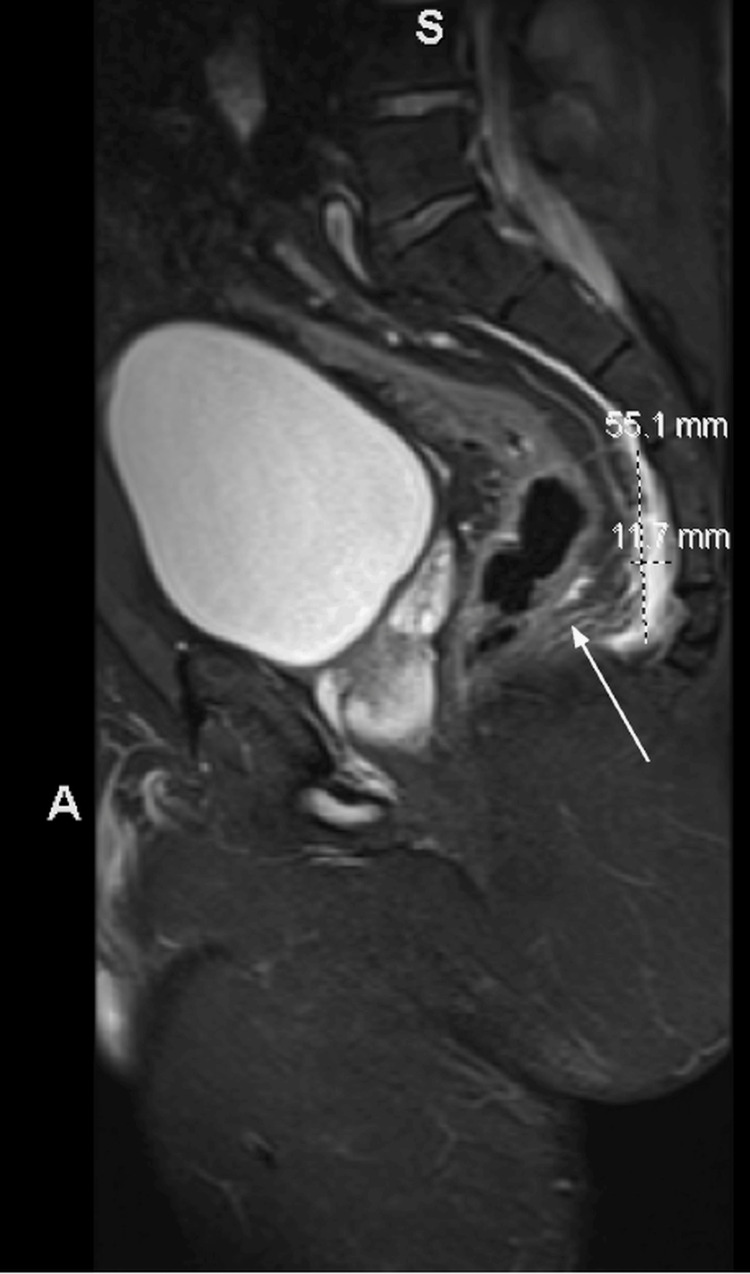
MRI of the pelvis with and without contrast showing the 5.5 x 1.2 x 5.7 cm presacral abscess formation. Of note, there was an intradural extension at the level of the left S1 and S2 neural foramen. Phlegmonous change over the lateral aspect of the left gluteus maximus muscle belly deep to the iliotibial band with small-volume abscess formation. No evidence of acute osteomyelitis.
